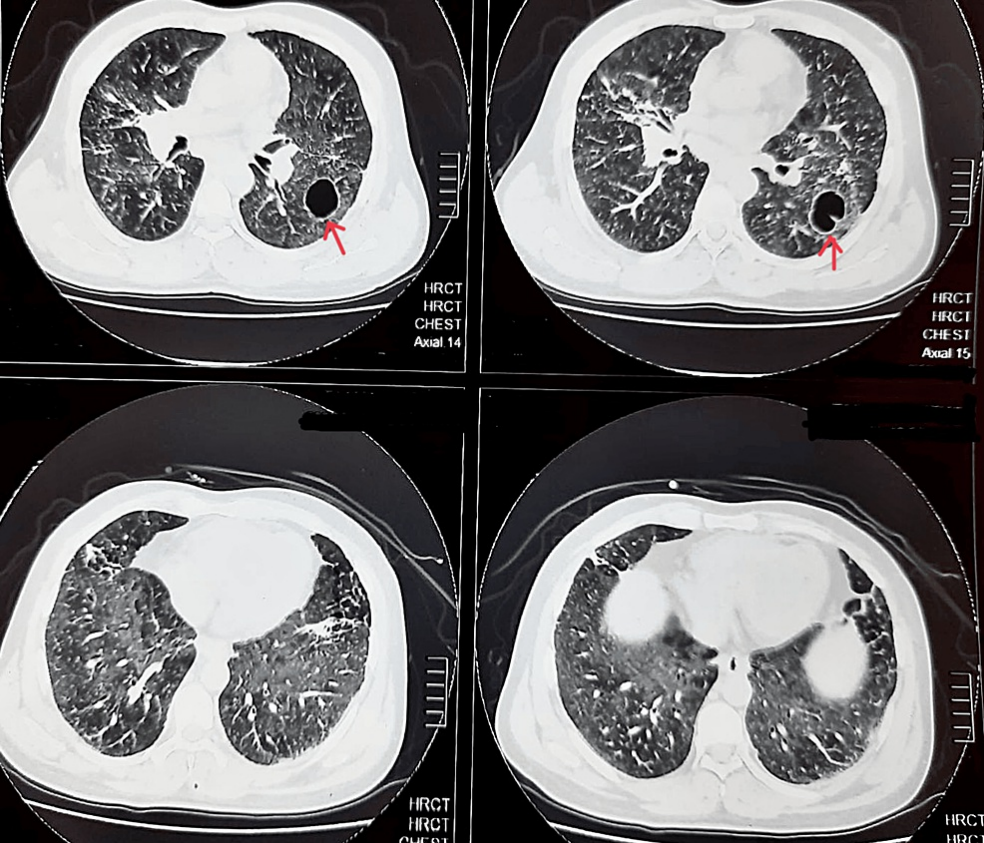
High-resolution computed tomography of the chest (axial view) showing cavitation, multiple pulmonary nodules, and interstitial thickening.
